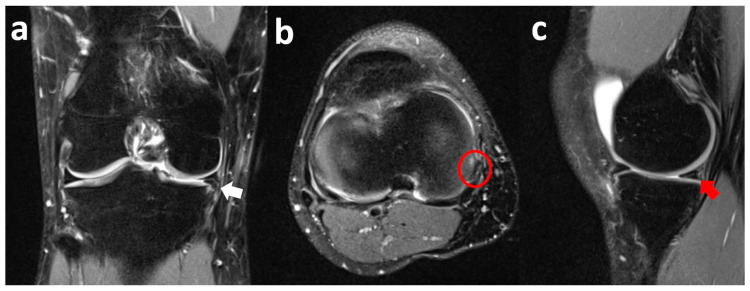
Right knee coronal fat-suppressed PD MR images (a) show a medial meniscus body tear with an open oblique course on the inferior side associated with a meniscal flap (white arrow) displaced inferiorly and trapped between the medial tibial bony surface and medial collateral and capsular–ligamentous complex. Axial image (b) shows the fragment at the level of the posteromedial tibial plateau (red circle). Sagittal images (c) show a normal appearance of the posterior horn (red arrow) with just a minimal defect at its free margin and a slightly hyperintense intrameniscal line.
